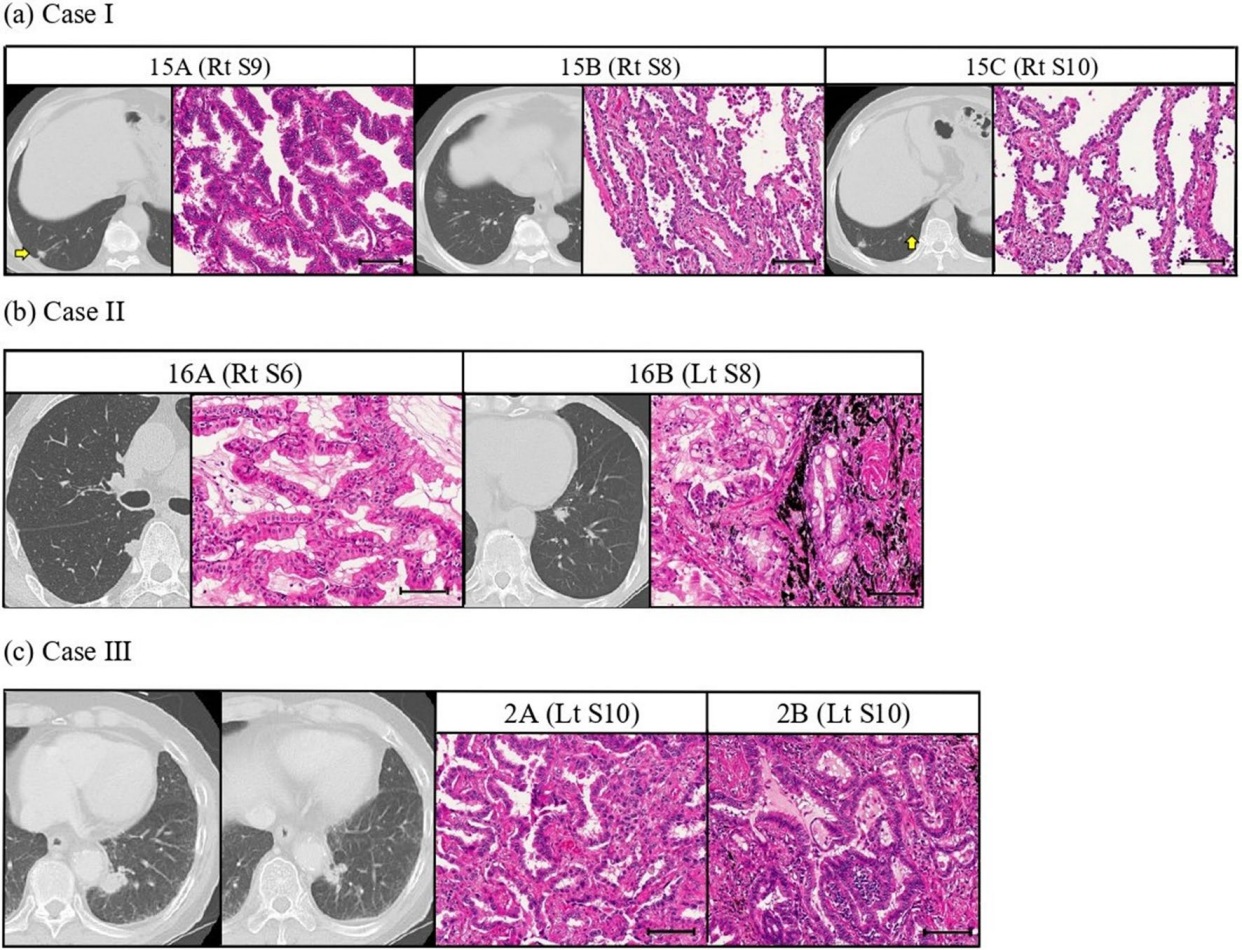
Summary of case according to radiological and pathological examination. (a) In case I, the tumours in right S8 and S10 were classified as adenocarcinoma in situ, and the tumour of right S9 were classified predominantly papillary adenocarcinoma. (b) In case II, the right S6 tumour was classified as AIS, and the left S8 tumour was classified papillary adenocarcinoma. (c) In case III, CT imaging showed two masses were adjacent to each other in the left S10. Both tumours were classified as predominantly papillary adenocarcinomas. However, the 2A tumour included lepidic construction and the 2B lesion contained relatively large amounts of acinar components without a lepidic component. Morphologic features were not completely similar between them. Scale bar = 100 μm. All figures were created using Paint 3D version 6.2009.30067.0, and those figures were edited to 300 dpi resolution using GIMP version 2.10.20.
